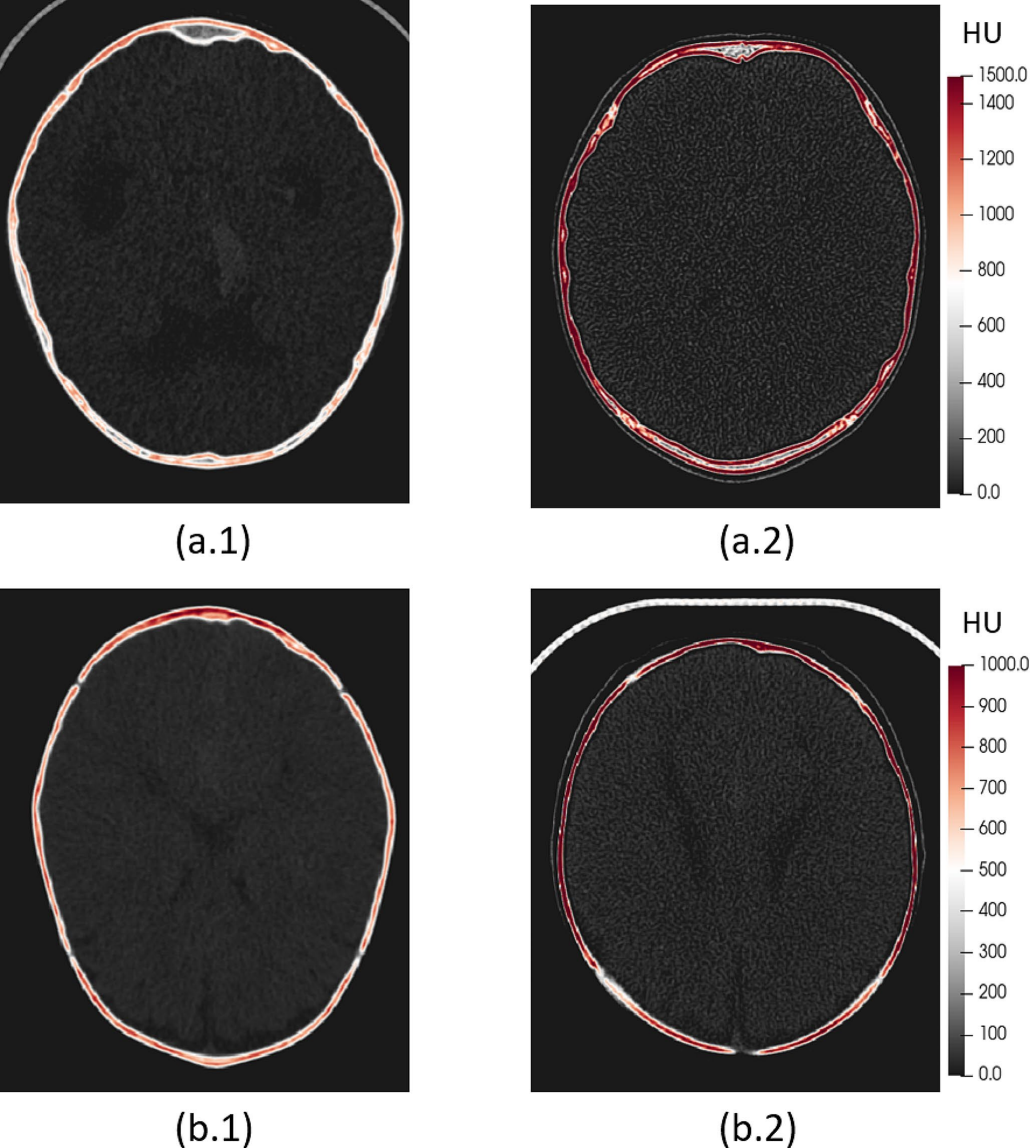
Qualitative demonstration of the bone anomalies in the axial CT image slices of patients with chronic IIP (a.1) and NSSC (b.1), compared to age- and sex-matched normative subjects (a.2 and b.2). (a.1) 7.7-year-old male patient with chronic IIP secondary to intracranial neoplasms and decreased calvarial bone density. (a.2) 7.7-year-old normative male subject. (b.1) 10.9-month-old female patient with untreated NSSC (b.1) and decreased calvarial bone density. (b.2) 10.9-month-old female normative reference (b.2). Cranial bone density in Hounsfield Units (HU) is represented color-coded
